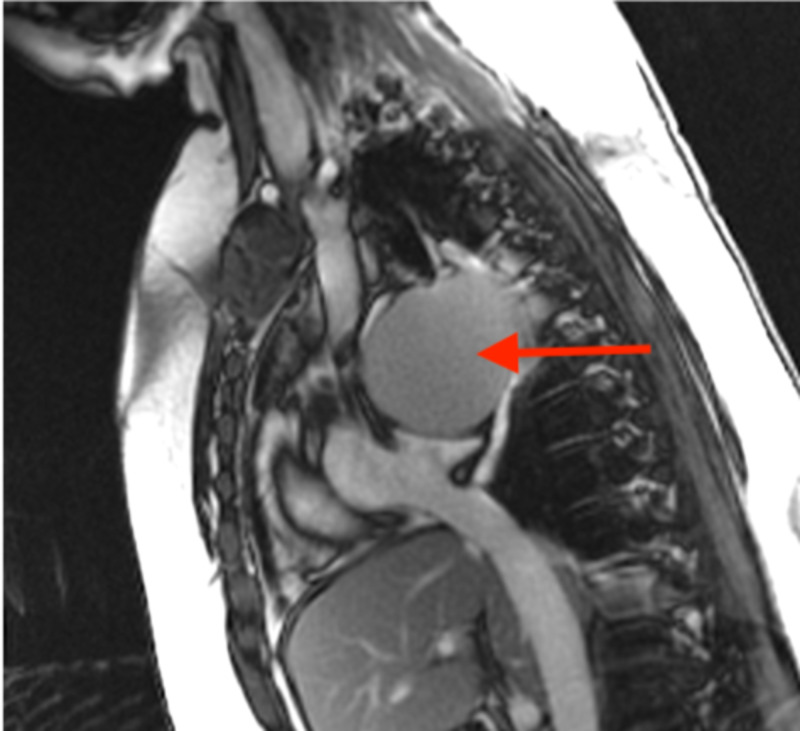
Cardiac MRI (FIESTA, RVOT view) showing an 8.2-cm subcarinal cystic lesion (red arrow) with uniform T2 hyperintensity, exerting mass effect on the left atrium. No large solid component is identified. FIESTA, fast imaging employing steady-state acquisition; RVOT, right ventricular outflow tract
